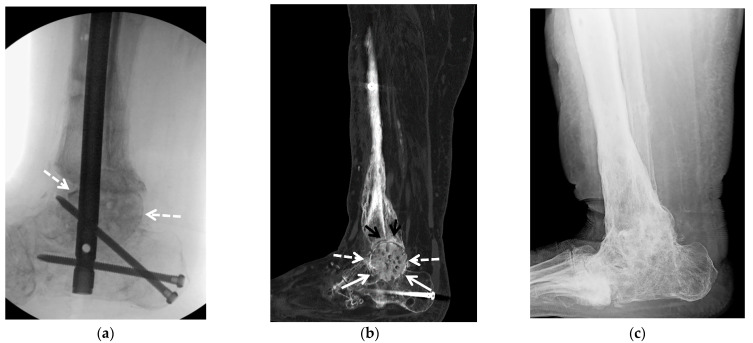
(a–c). A 58-year-old male with a complex medical history including diabetes mellitus, infected right Charcot midfoot and hindfoot as well as advanced secondary osteoarthritis, treated with staged fusion: first external fixation with antibiotic spacer and intravenous antibiotics followed by tibiotalocalcaneal fusion with a structural femoral head allograft. (a) Fluoroscopic lateral intraoperative image of the right ankle shows a retrograde tibiotalocalcaneal intramedullary nail with two distal screws in the calcaneus and across the posterior calcaneal facet, and a structural femoral head allograft placed at the talar dome bone void and resection site (white arrows). (b) Sagittal reformatted CT image obtained 3.5 months after surgery shows the structural femoral head allograft (dashed white arrows) replacing the talar dome, between the calcaneus (white arrows), talar head and tibial plafond (black arrows). Partially visualized is tibiotalocalcaneal fixation hardware. (c) Seven months postoperative lateral weight-bearing radiograph status post hardware removal shows complete solid bony fusion across the subtalar and tibiotalar joints with complete incorporation of the structural femoral head allograft.
